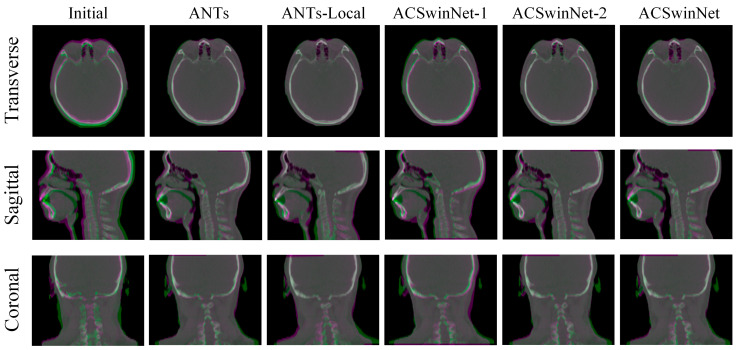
A visual comparison of the image registration result of images with slight non-linear deformation in the neck area is presented. For better observation, CT and CBCT images are colored in magenta and green, respectively, while the overlapping region is shown in gray. Results are displayed in the view of the transverse, sagittal and coronal sections. The first column shows the fused image before registration, whereas the other columns show the fused images of results produced by different methods.
